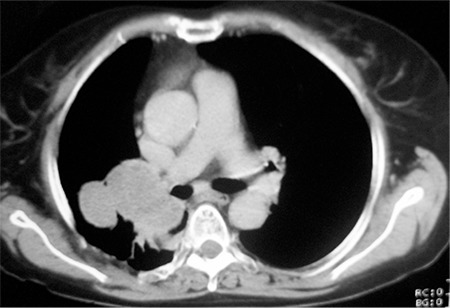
CT scan of the chest showing a lobulated mass with heterogeneous enhancement, compressing the mediastinum, right main bronchus and right pulmonary artery.
